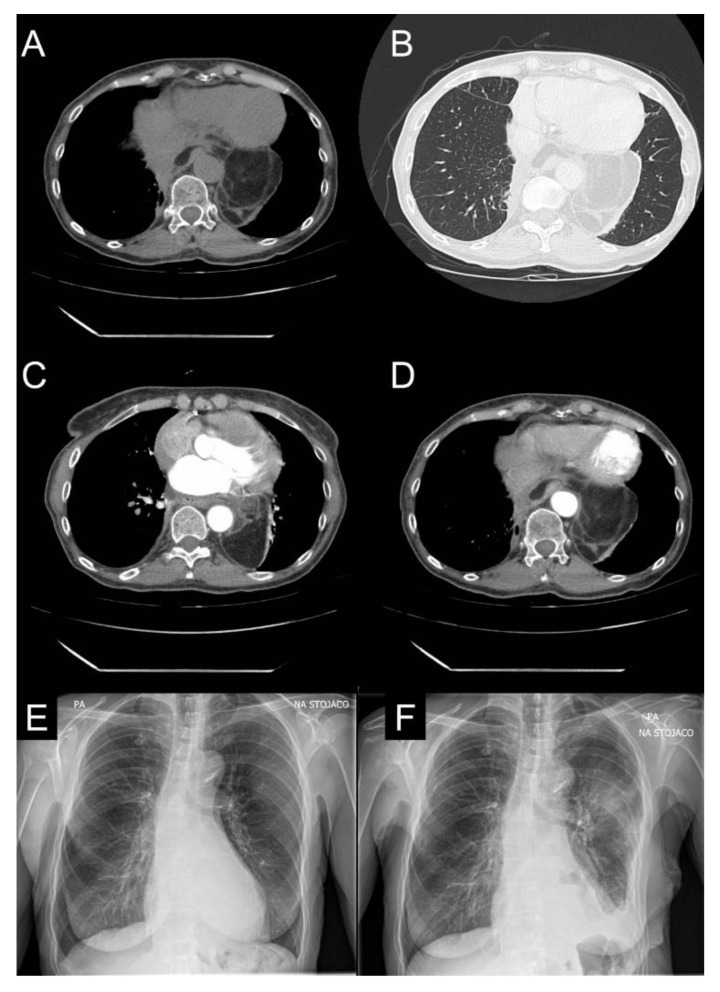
Radiological imaging of the patient during the second surgery. (A–D): CT images from August 2022 with a massive lesion in the posterior mediastinum. (E,F): Pre- and postoperative chest X-rays of the patient from 21 September 2022 and 29 September 2022, respectively.
